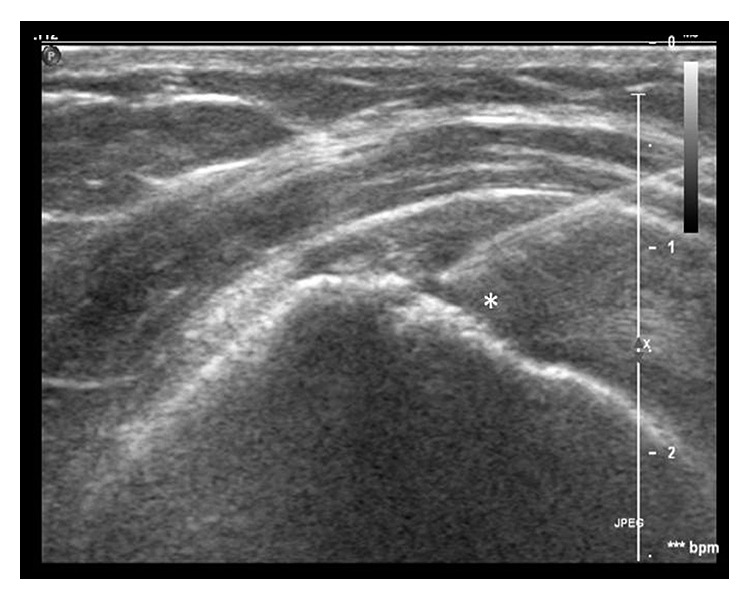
Longitudinal sonographic image obtained using a 5–17 MHz linear array transducer after insertion of a 21-gauge needle shows the tip of the needle located in the area of tendinosis with prolotherapy injected (^*∗*^).
